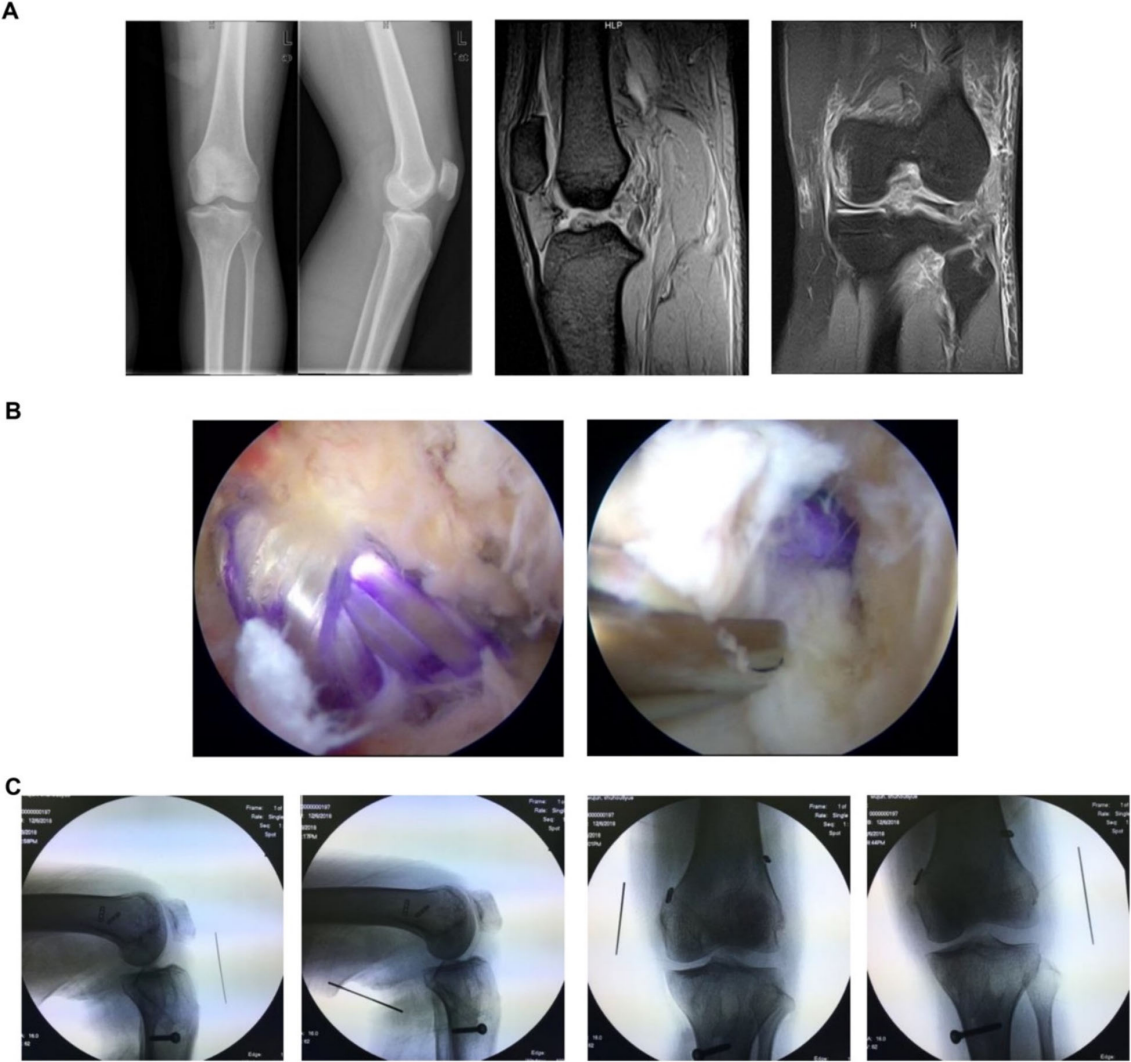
(A-C): A 48-year-old male patient suffered movement obstruction for 18 days after being hit by a heavy object on the superior part of the left knee. The whole diagnosis was acute stage KD-III: ACL + PCL + LCL, no fracture or cartilage injury, and accompanied by medial and lateral meniscus injury (A). The surgery was performed including arthroscope probing in left knee + ACL/PCL reconstruction + LCL repairing + medial and lateral meniscus repairing (B). An X-ray stress examination showed that the knee maintained good stability at the last follow-up (C). Functional scores after 1 year were International Knee Documentation Committee score: 75; Lysholm score: 83; and Tegner score: 4
